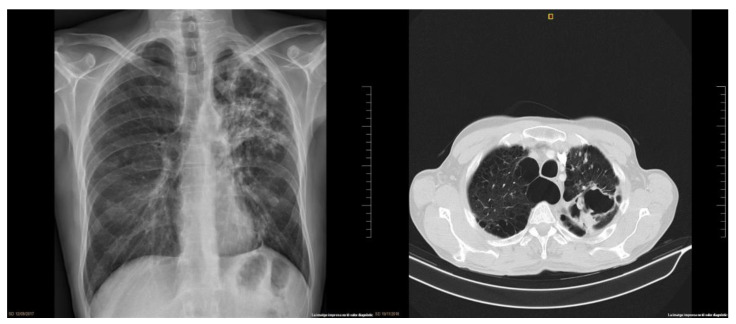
Lung images: (1) lung chest X-ray at diagnosis shows a left apical cavitation and surrounding infiltrate; (2) lung chest CT scan after clinical impairment shows central emphysema, fibrosis in both upper lobes with significant loss of volume, apical left lobe and diffuse bronchiectasis and a large apical cavity in left lung of approximately 7.1 cm.
